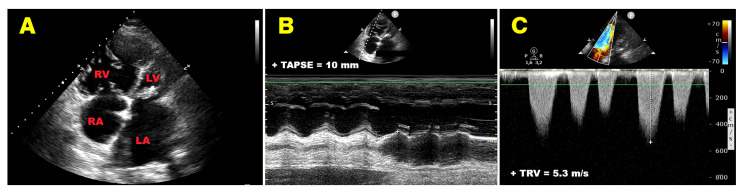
Representative example of severe right ventricular–pulmonary arterial (RV–PA) uncoupling in acute decompensated heart failure. (A) Transthoracic echocardiographic apical four-chamber view demonstrating marked right-sided chamber enlargement in a 60-year-old woman with severe rheumatic mitral stenosis and advanced congestive heart failure. (B) M-mode assessment of tricuspid annular plane systolic excursion (TAPSE), showing severely reduced right ventricular longitudinal systolic function (TAPSE = 10 mm). (C) Continuous-wave Doppler interrogation of the tricuspid regurgitant jet revealing markedly elevated tricuspid regurgitation velocity (TRV = 5.3 m/s), consistent with severe pulmonary hypertension. The combination of impaired right ventricular contractility and markedly increased pulmonary arterial afterload resulted in a profoundly reduced TAPSE/sPAP ratio (≈0.10 mm/mmHg), reflecting advanced RV–PA uncoupling and a high-risk hemodynamic profile.
